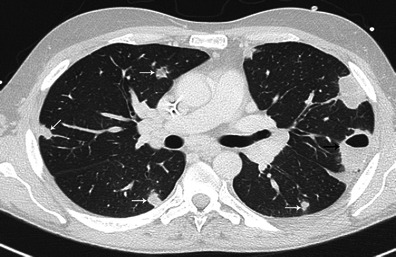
Thoracic enhanced CT shows a peripheral cavitary lesion (*black arrow*) with numerous lung nodules (*white arrows*) complicating the evolution of right side infective endocarditis
